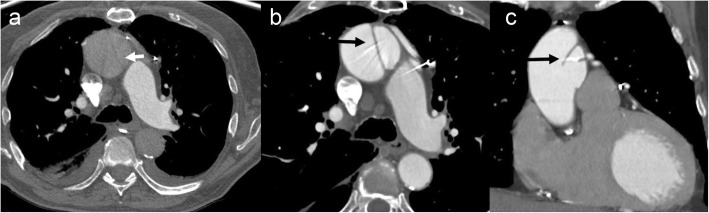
A 75-year-old man with shortness of breath and chest pain that underwent high-pitch CTA pulmonary artery (a) that was negative for pulmonary embolism but a concern for aortic dissection was raised by the cardiovascular radiologist. Aortic protocol CTA confirmed a type A dissection with entry point adjacent to coronary bypass graft (arrow in b and c) that was very subtle on the CTA pulmonary artery (a)
